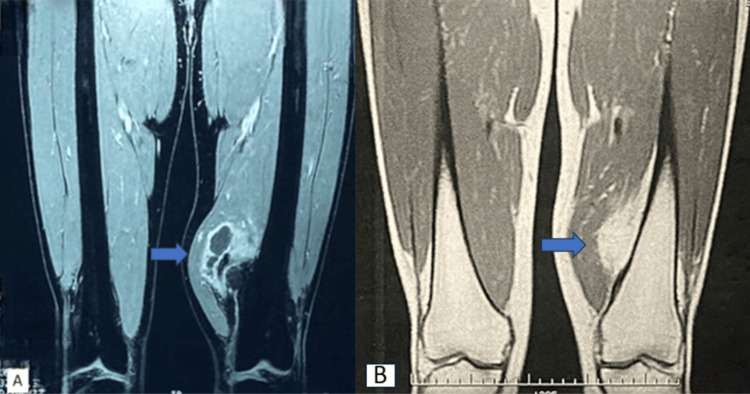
MRI of the left thigh showing a well-limited mass in the anterior compartment of the thigh, in continuity with the medial cortex of the femur, displacing the vastus medialis; hypointense in T1 (A) and hyperintense in T2 (B) with peripheral reassessment.
